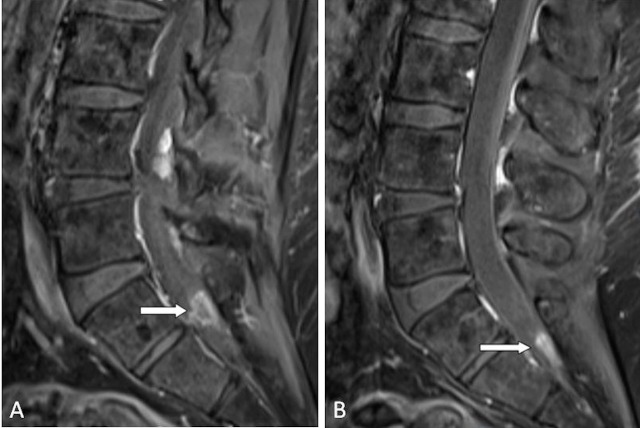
Preoperative MRI Spine Parasagittal gadolinium enhanced T1-weighted MRI demonstrating an intradural lesion at the level of S1-2 abutting the left S2 nerve root (A) and at the level of S2 (B).
